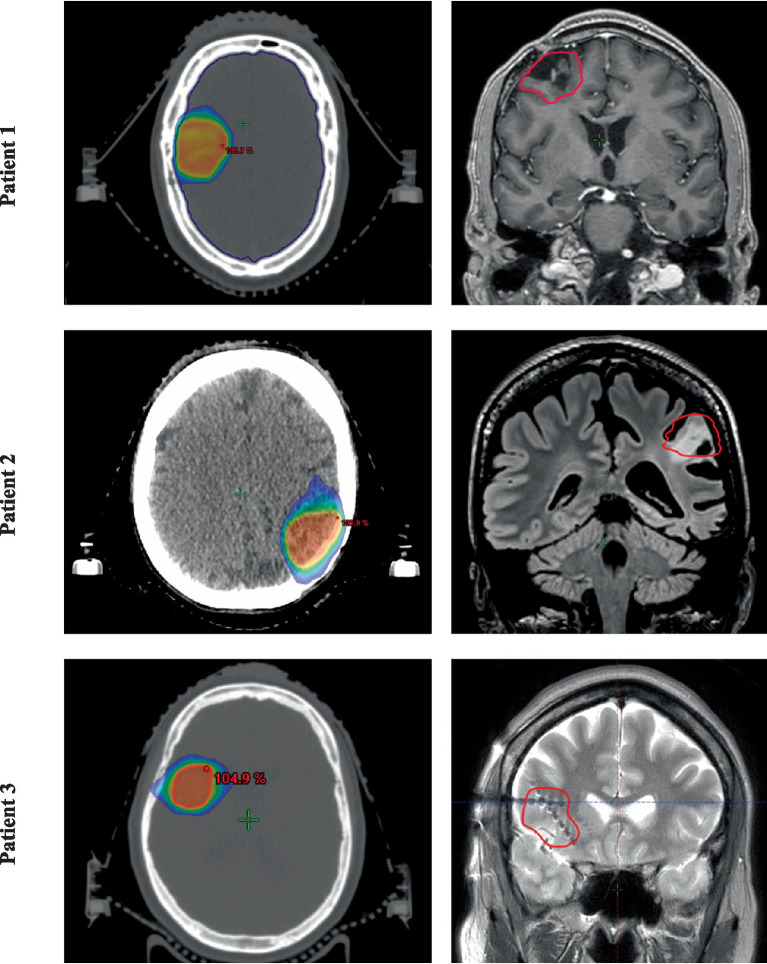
Target volume and treatment planning for all three patients. (Left) Axial fractionated stereotactic radiotherapy plan, showing the 50% isodose line for all three patients. (Right) Superimposed coronal brain MRI used to accurately define the target volume (red).
